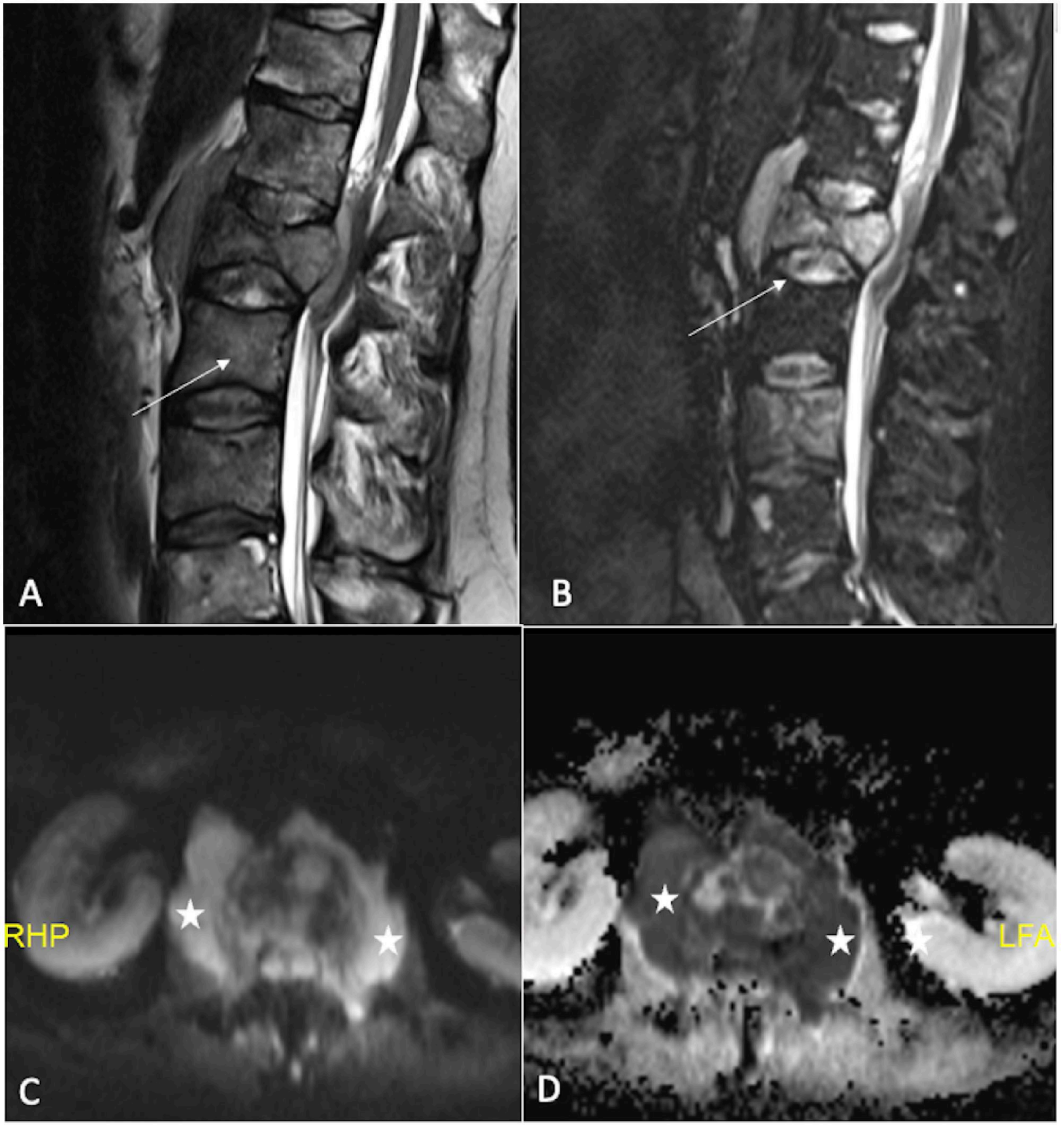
Bone lymphoma. A 45-year-old male showed multiple osteolytic lesions affecting several vertebral bodies, accompanied by compression collapse and associated soft tissue components (indicated by arrows in A and B). DWI shows hyperintense signal (marked by stars in C) in the soft tissues, with a corresponding marked ADC restriction (ADC = 0.6×10^−3^mm^2^/s), typically seen with round cell tumours (also marked by stars in D).
